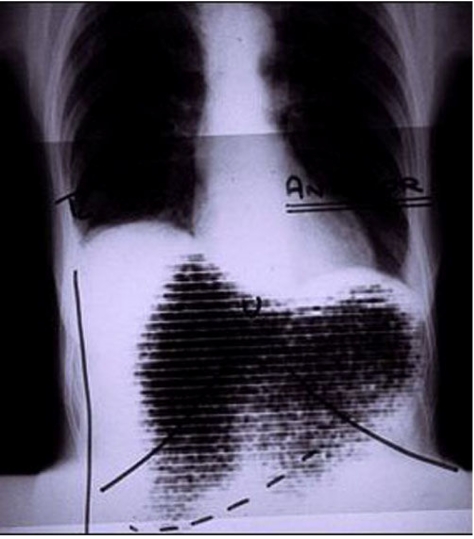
A fusion of a rectilinear scan using I-131 rose Bengal with a chest X-ray outlining the position of the diaphragm was used to diagnose subphrenic abscess before the availability of CT scanning and ultrasound. This represented one of the first examples of “anatamometabolic” imaging.
